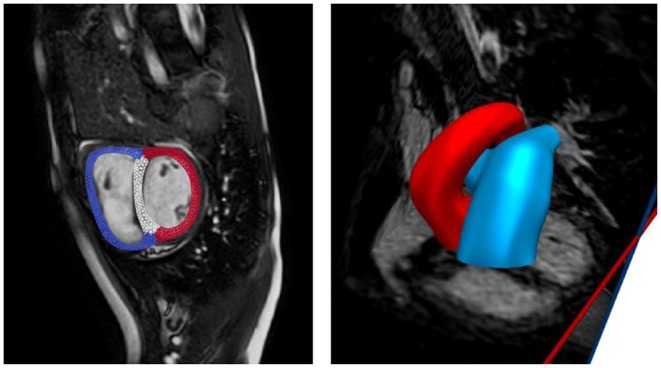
Reconstruction of biventricular model (left) and large proximal arteries (right) from cine MR images.
